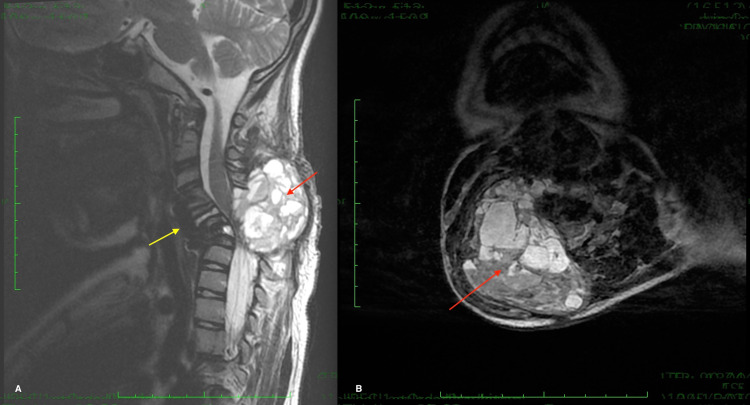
Sagittal (A) and axial (B) MRI showing posterior tumor growth with spinal cord compression, right paravertebral soft tissue extension (red arrow), and the anterior fixation by C4-C6 plate and cervical kyphosis (yellow arrow)
